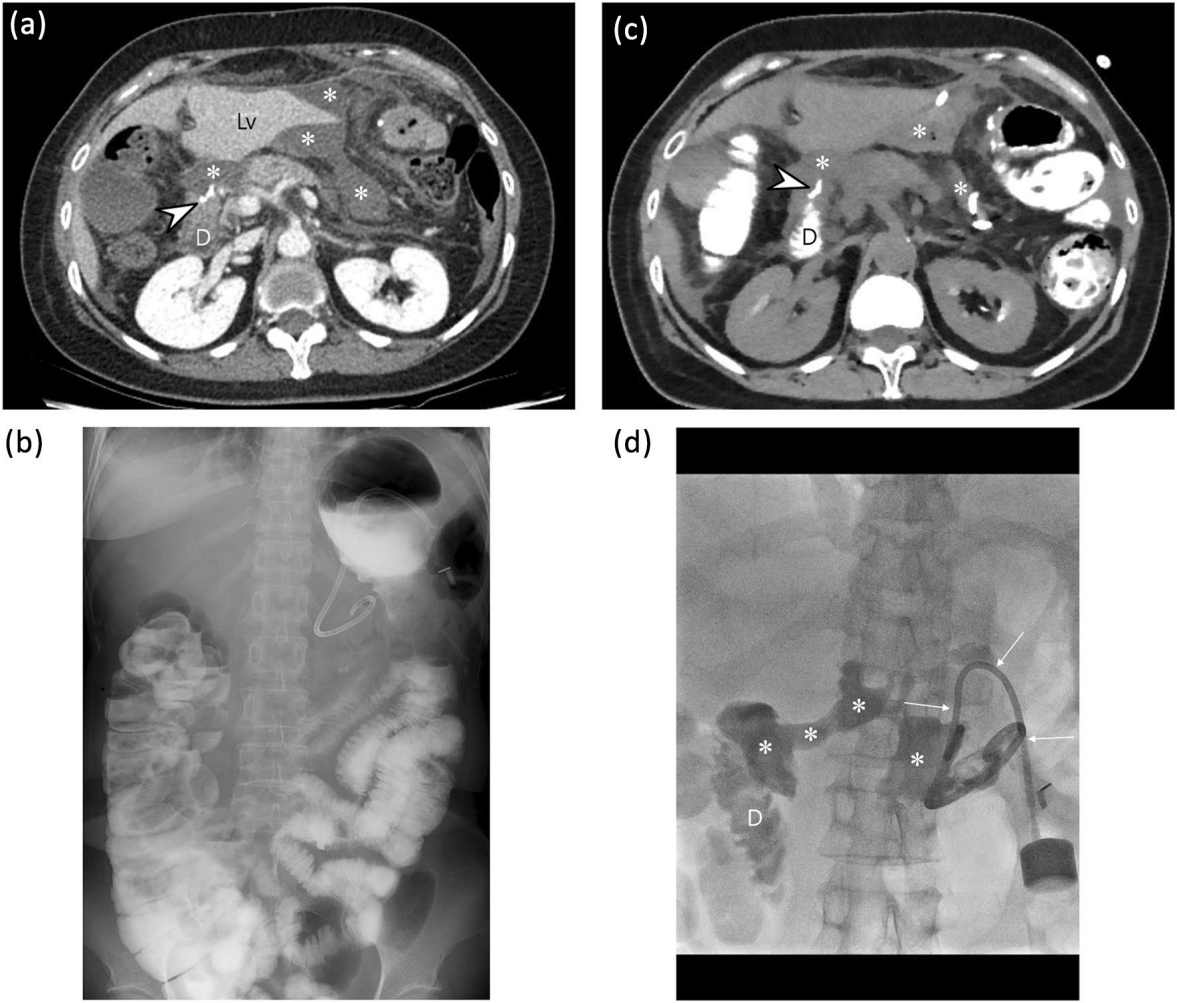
A 49-year old woman who underwent distal gastrectomy with Billroth II anastomosis for early gastric cancer. Ten days after the operation, the patient visited an emergency department due to severe abdominal pain. (a) On portal phase contrast-enhanced CT, multiple fluid collection (*) around the duodenal stump (D) and the liver (Lv) is observed. Therefore, leakage at the duodenal stump was highly suspected. (b) On a delayed fluoroscopic image obtained during upper gastrointestinal series using 200 ml of a 1:1 diluted enteric iodinated contrast agent (gastrografin®), there is no definite leakage. Two radiologists scored this patient as 1 (definitely absent) for the presence of leakage. (c) On an axial non-contrast CT image obtained immediately after fluoroscopic examination, there is no definite contrast extravasation around the duodenal stump (D) or within several fluid collections (*). Therefore, both radiologists scored this patient as 1 (definitely absent) for the presence of leakage. (d) On a tubogram obtained after contrast injection through a percutaneous catheter drainage (PCD) tube (thin arrows), the duodenal 2nd portion (d) is opacified, along with multiple fluid collection (*). Therefore, duodenal stump leakage was finally diagnosed. This is a case of false-negative leakage on both fluoroscopy and positive enteric contrast CT.
